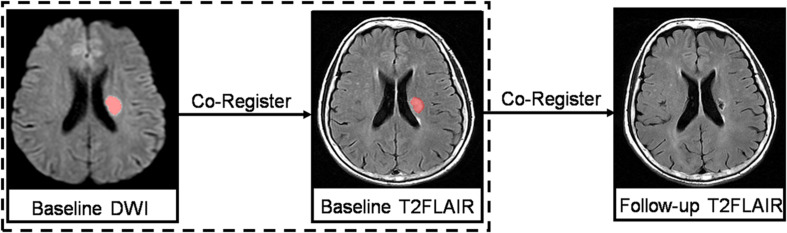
Baseline DWIs were registered to the baseline and follow-up T2 FLAIR images to ensure observation of the same lesion.
